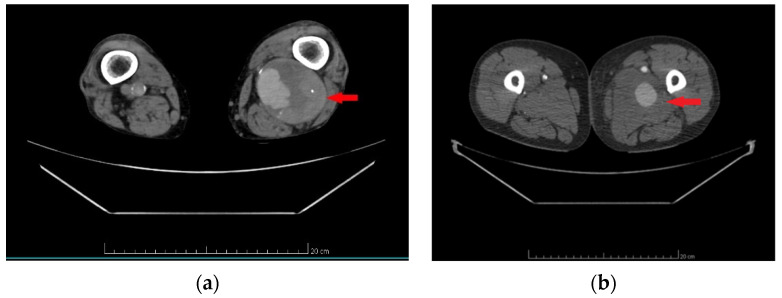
Computed tomography angiography—true aneurysm of popliteal artery (a), pseudoaneurysm of common femoral artery (b). Note: (a) (red arrow)—round, sharply demarcated from the surrounding tissues dilatation with the involvement of the intima, media, and adventitia of the popliteal artery. (b) (red arrow)—poorly demarcated common femoral artery dilations contained by periarterial connective tissue.
